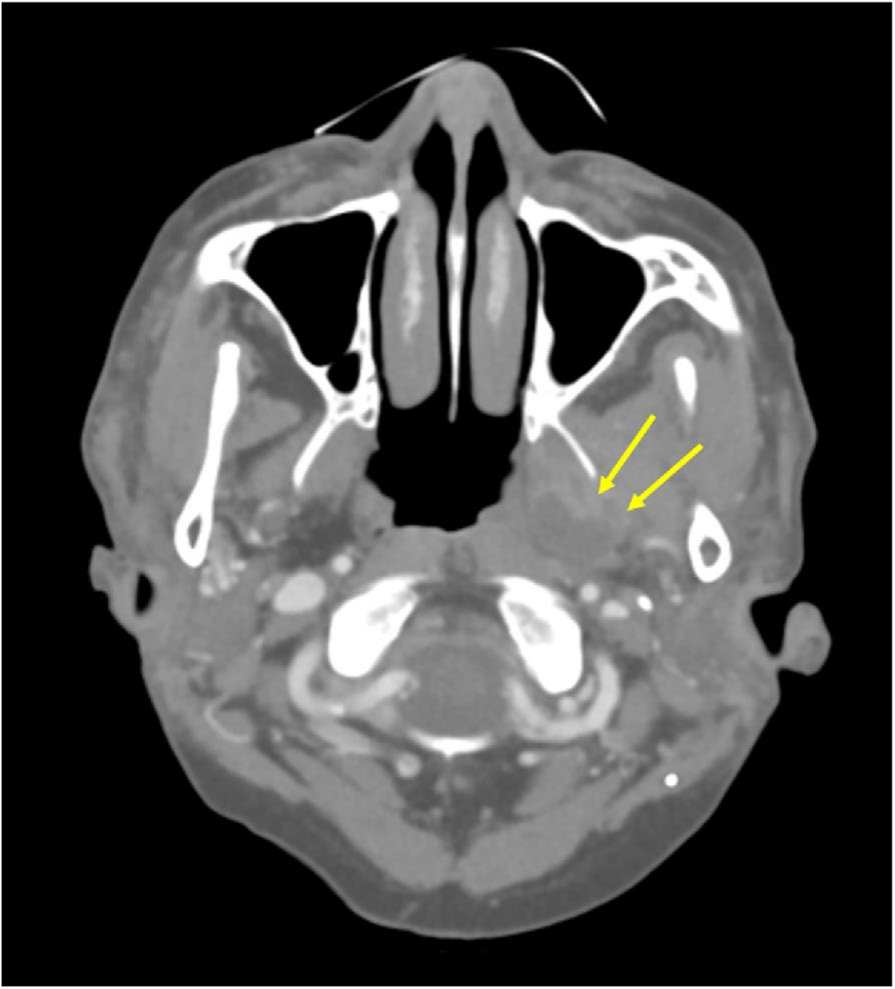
Enhanced CT image at 7 days after the first visit (case 3). Heterogeneous lesions of the left pterygoid and parapharyngeal space (yellow arrows) were observed
